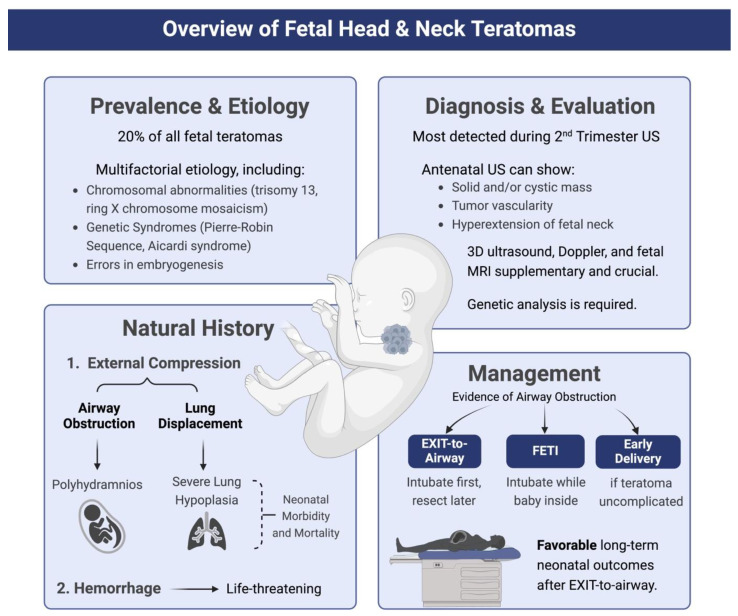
Overview for diagnosis and management of fetal head and neck teratomas. US: ultrasound; EXIT: ex utero intrapartum treatment; FETI: fetal endoscopic tracheal intubation.
